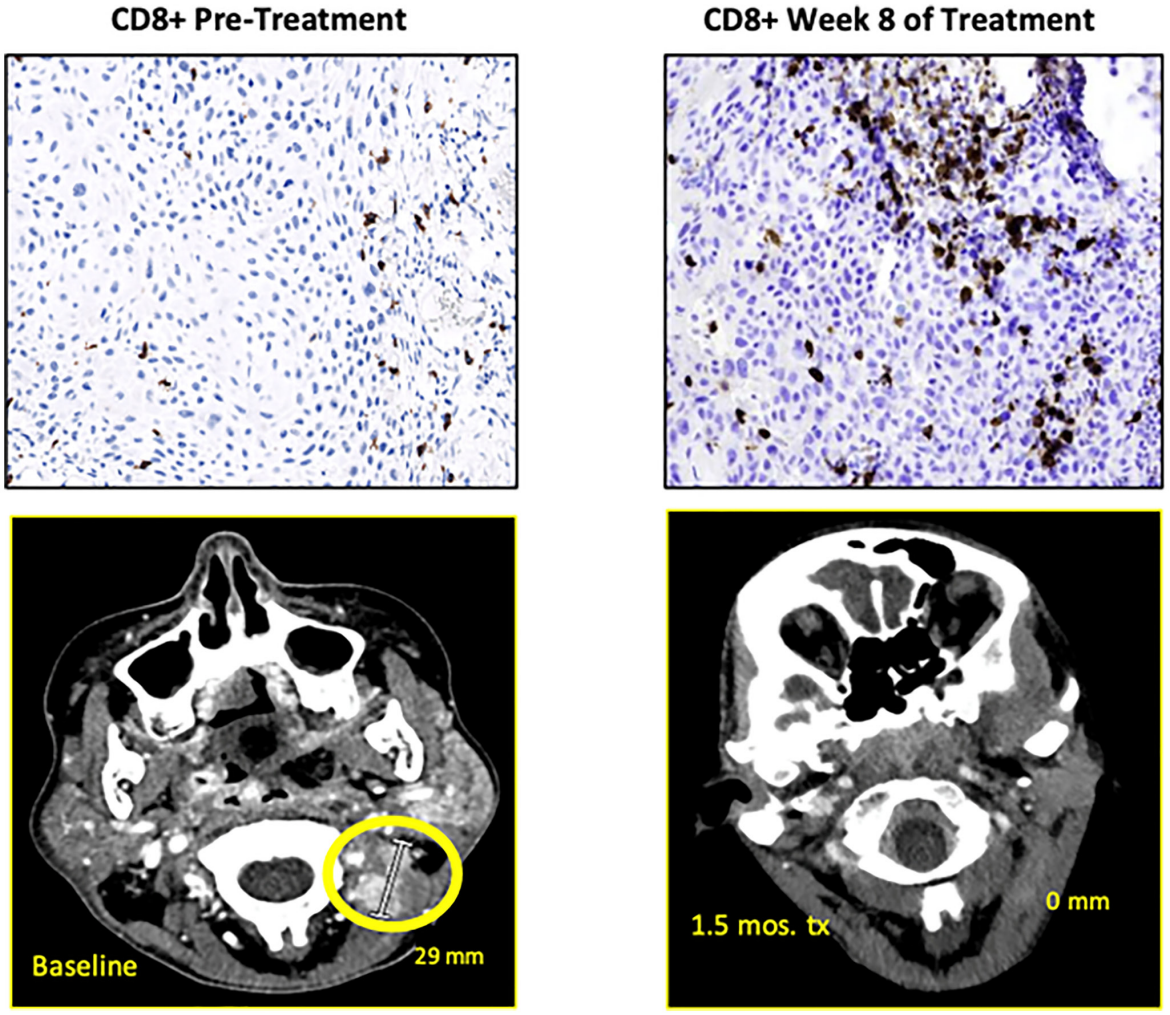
Baseline and on-therapy imaging and biopsy results showing radiographic resolution of tumor and increase of intratumoral COB+ cells.
